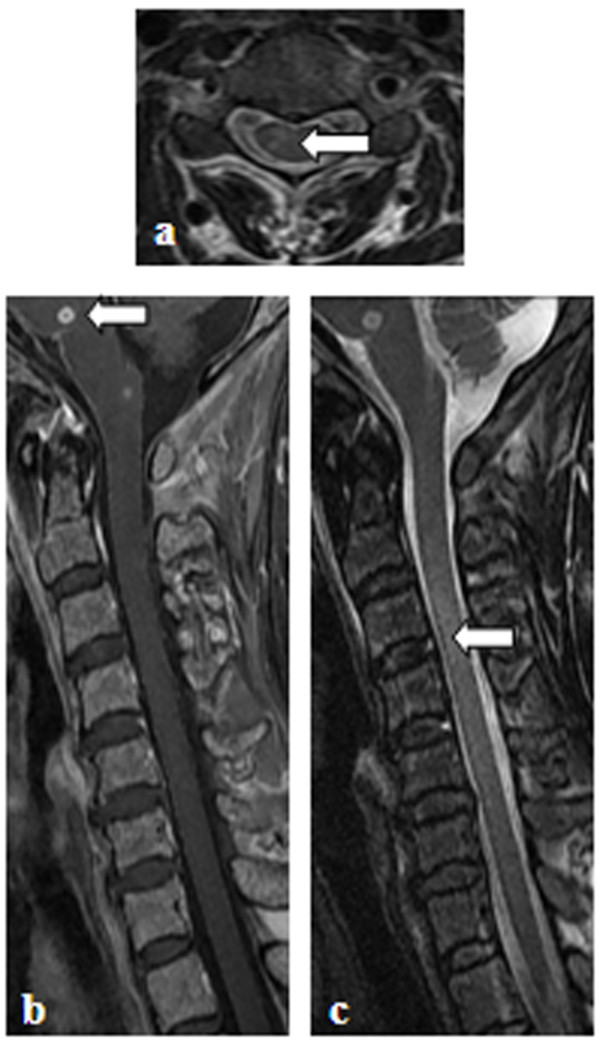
Spinal MRI of a CNS-TB patient with spinal cord involvement. a: axial T2-weighted image showing central gray matter involvement of the spinal cord. b: T1-weighted contrast image revealing tuberculoma enhancement in the medulla but no enhancement of the spinal cord lesion; c: T2-weighted image showing a longitudinal extensive spinal cord lesion without a well-demarcated boundary.
